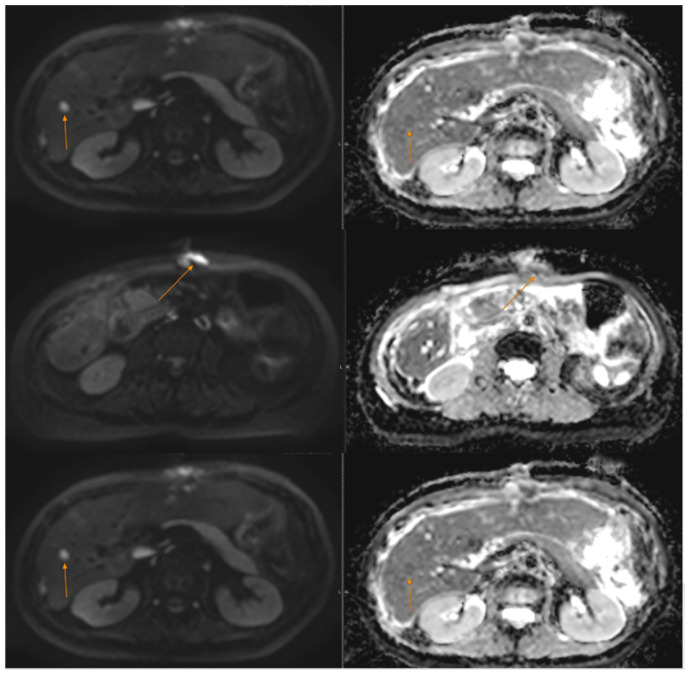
Liver and peritoneal metastases on MRI post-cito-reductive surgery.
